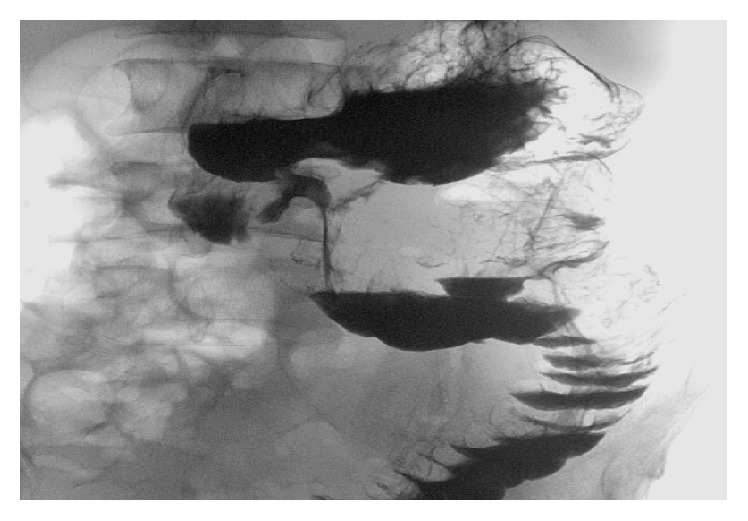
Barium X-ray confirming the GCF.
